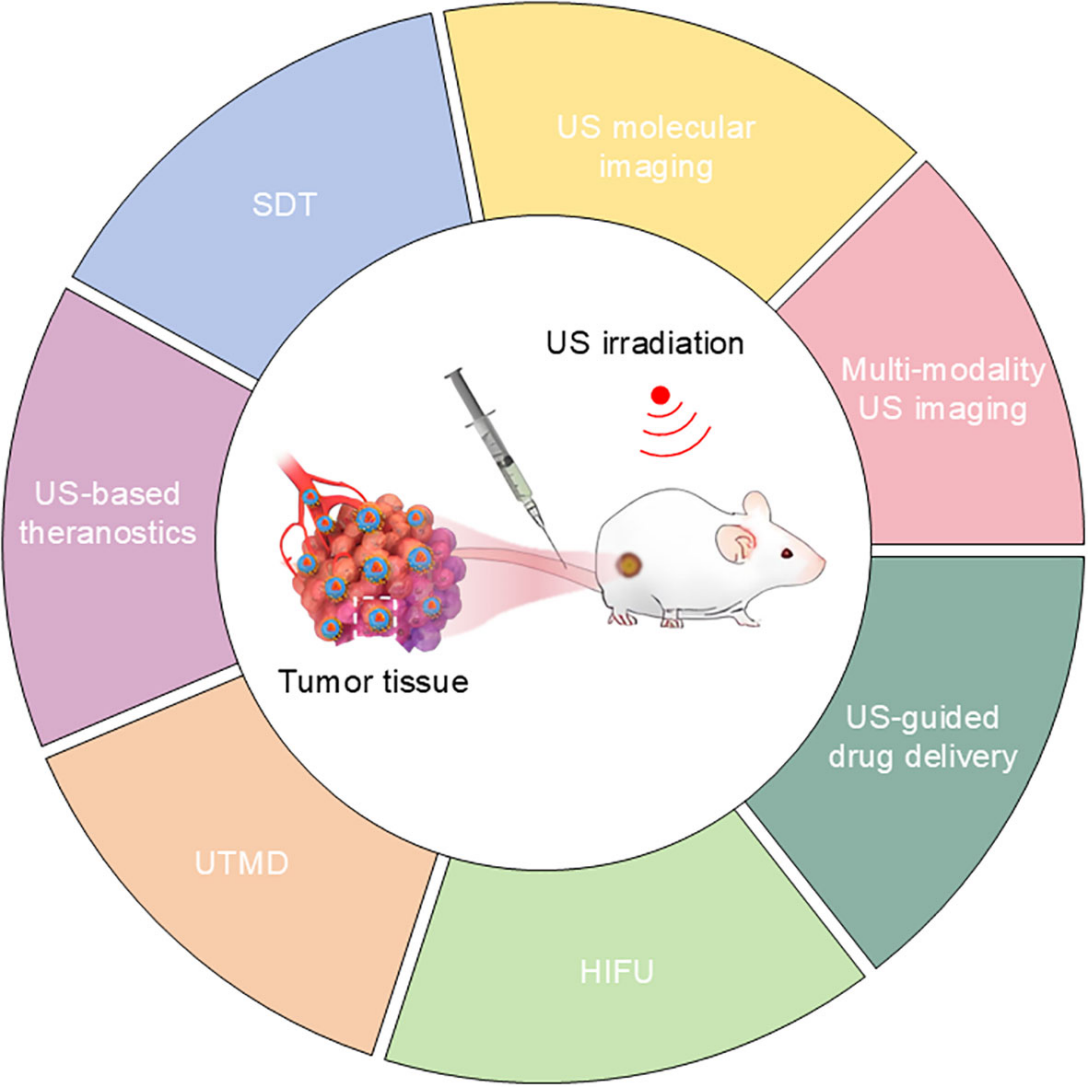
Nanoplatform-based ultrasound application structural framework.
